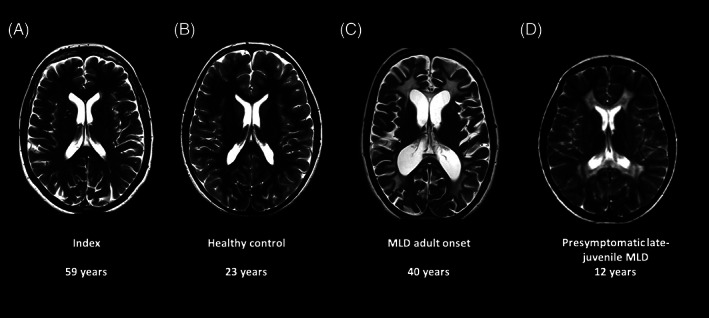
MRI data. Cerebral T2‐weighted MRI of the index case at the age of 59 years (A) with no signs of demyelination, which is comparable to that of a healthy adult control (B). In contrast, T2‐weighted MRI of a patient with adult onset of MLD (C) and juvenile onset of MLD (D) reveal marked demyelination
